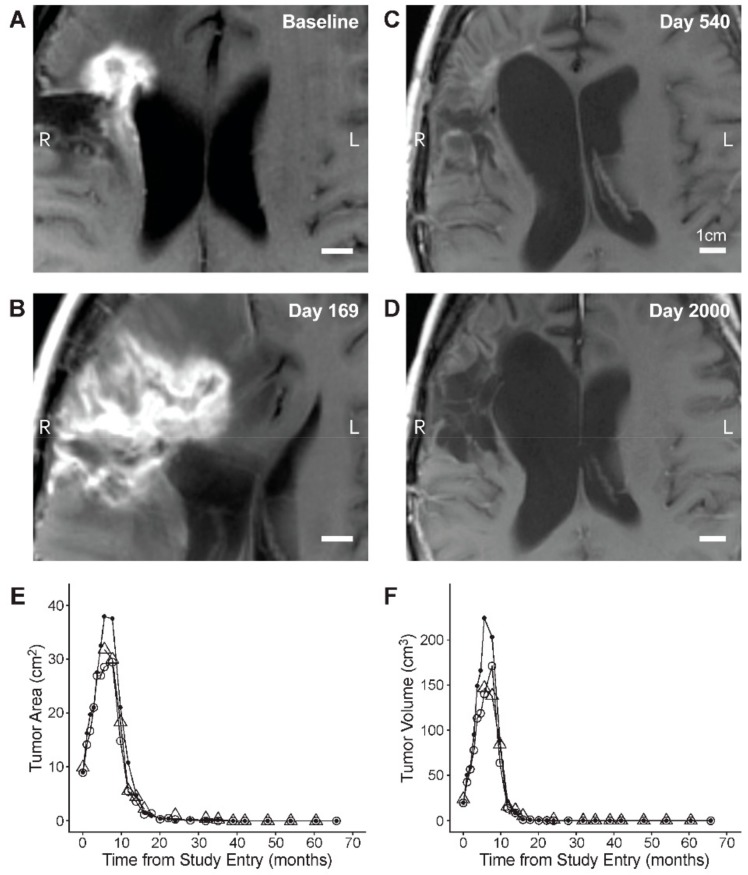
Pseudo-progression and CR in *R/R* AA (WHO Grade 3) patient, UPN203302. (A–D): Depicted are T1-weighted spin echo (SE) axial MRI images obtained at baseline and at the indicated time points after randomization to the 2.5 mg/cycle dose cohort of OT101. See Figure S6 for details provided for the same images in smaller magnification. (E and F): Review of MRI images by two-to-three independent reviewers (open circle: Reviewer 1; closed circle: Reviewer 2; triangle: Reviewer 3/adjudicator) showed a time-dependent decrease of the 2-D (Panel E) and 3-D (Panels F) size of the target lesion following a significant increase during the early period of pseudo-progression.
